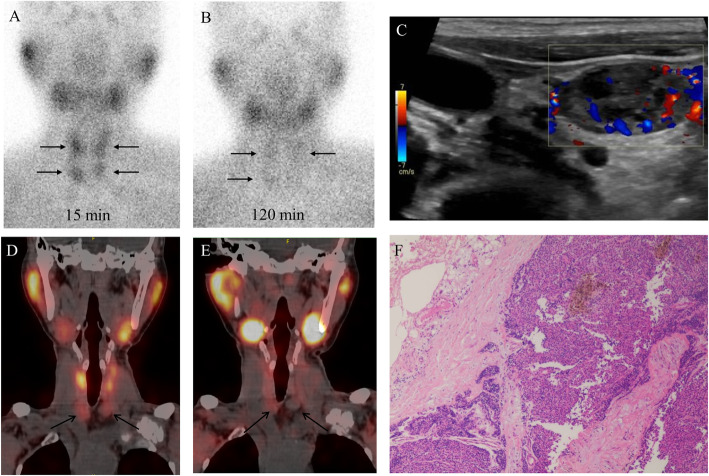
Representative images of a middle-aged patient with SHPT. Dual-phase ^99m^Tc-MIBI scintigraphy (A and B) showed 4 hyperplastic parathyroid glands. US (C) showed a typical enlarged parathyroid gland on the right. Early SPECT/CT (D) showed right inferior and left inferior hyperplastic parathyroid glands with ^99m^Tc-MIBI uptake. Delayed SPECT/CT (E) showed right inferior hyperplastic parathyroid gland with ^99m^Tc-MIBI uptake and left inferior hyperplastic parathyroid gland without ^99m^Tc-MIBI uptake. Pathological examination (F) confirmed nodular parathyroid hyperplasia
